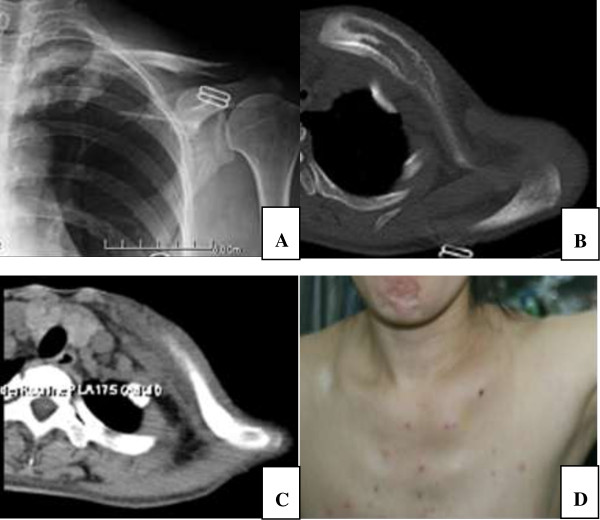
A 31 years female patient with bone lymphoma of left clavicle.Iconography shows bone expansion and soluble bony destruction. The main symptoms are swelling and pain. A. Preoperative X-film shows osteolytic destruction of left clavicle. B, C. Preoperative CT scan show the range of lesion, no subclavian vein compression. D. Outside view of shoulder, main sign is swelling.
